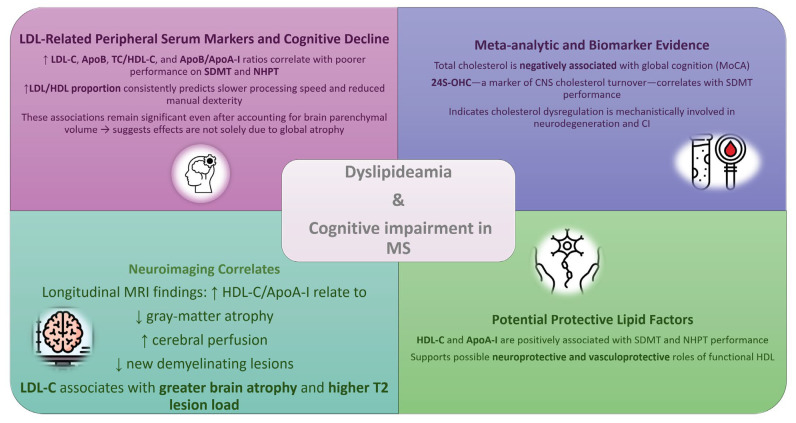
LDL-related markers link to poorer cognitive and motor performance, while cholesterol-turnover biomarkers and MRI measures indicate structural correlates of cognitive decline. Functional HDL (HDL-C, ApoA-I) may exert protective effects. Abbreviations: LDL-C—low-density lipoprotein cholesterol; HDL-C—high-density lipoprotein cholesterol; ApoA-I/ApoB—apolipoproteins A-I and B; TC—total cholesterol; 24S-OHC—24-S-hydroxycholesterol; SDMT—Symbol Digit Modalities Test; NHPT—Nine-Hole Peg Test; CI—cognitive impairment,↑—increased, ↓—decreased.
